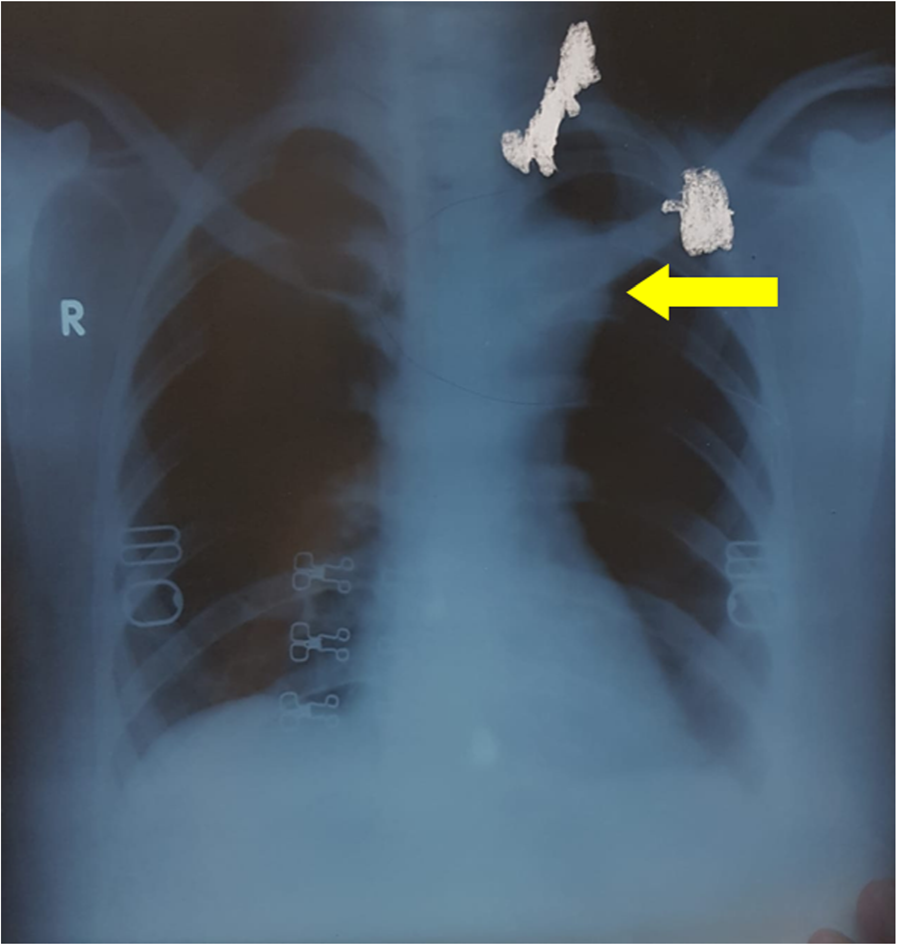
Chest Xray PA view showing widened mediastinum (yellow arrow)
